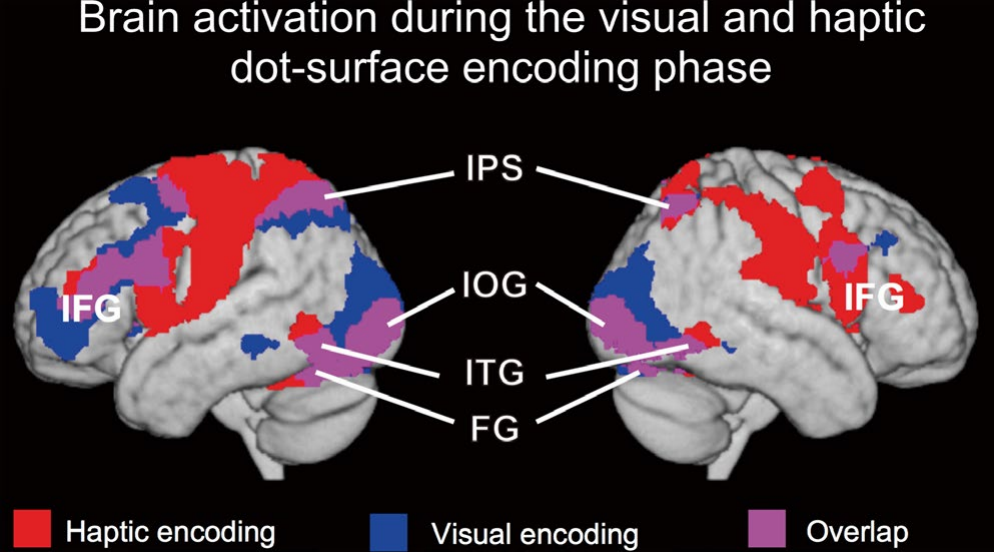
Brain activation during the visual and haptic dot‐surface encoding phase. Areas of significant activation are rendered on a normalized T1‐weighted high‐resolution brain MRI averaged across 18 subjects and the axial section of the same image. The extent threshold for activation was *p* < .05, corrected for each search volume with a height threshold of *T* (68) = 3.21 (equivalent to *p* < .001, uncorrected). FG, fusiform gyrus; IFG, inferior frontal gyrus; IOG, inferior occipital gyrus; IPS, intraparietal sulcus; ITG, inferior temporal gyrus
